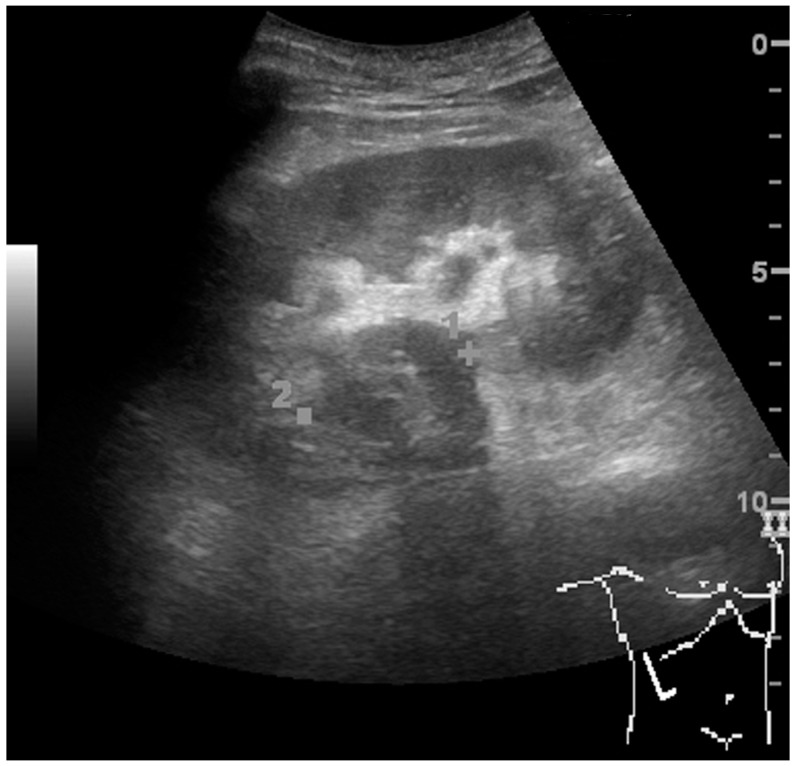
Solid tumor in the renal sinus seen as a hypoechoic mass, later found to be lymphoma. The ‘1’ and ‘2’ on the US image are reference points used for CT fusion (not shown).
